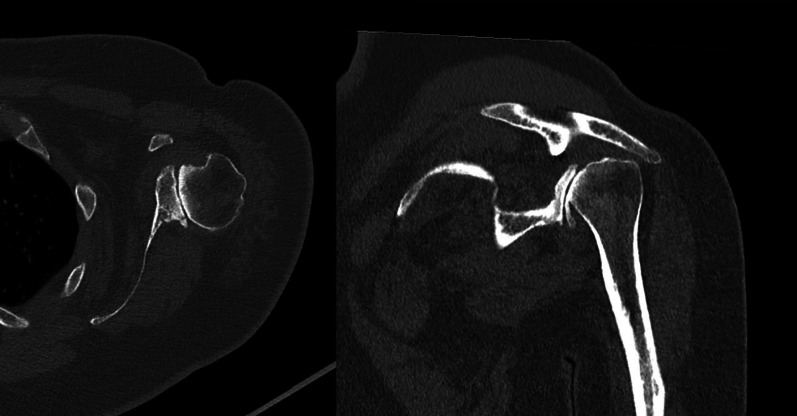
Axial and Sagittal CT scan views showing retroversion of glenoid
